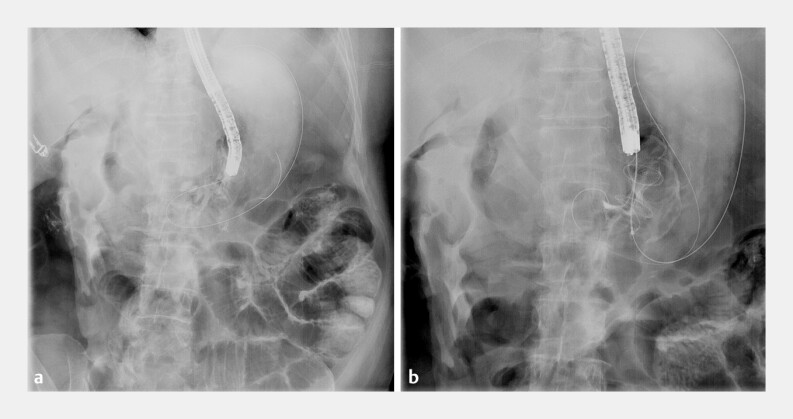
Radiographic images of the lumen-apposing metal stent (LAMS)-in-LAMS technique being performed over a guidewire showing:
